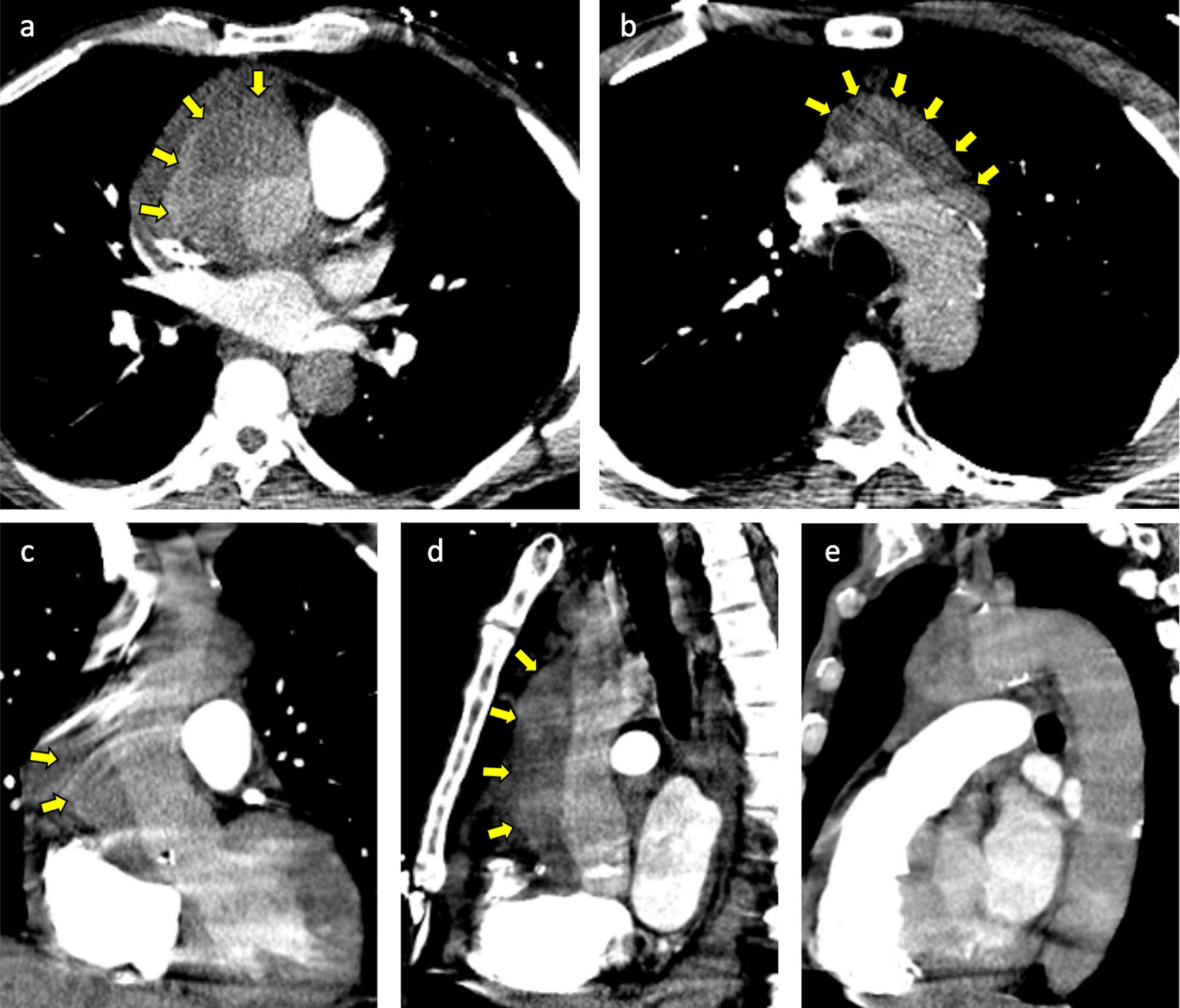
Preoperative computed tomography shows Stanford type A aortic dissection of the ascending aorta and the aortic arch (arrows). There was no dissection in the descending aorta. (a) Transverse view of the ascending aorta, (b) transverse view of the aortic arch, (c) coronal view of the ascending aorta, (d) sagittal view of the ascending aorta, and (e) sagittal view of the descending aorta
